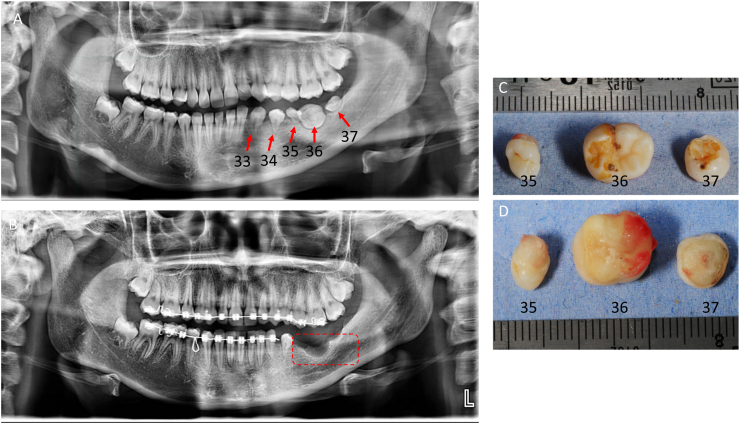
Panoramic radiograph showing abnormal development of teeth 33, 34, 35, 36, and 37, with notable defects in enamel and dentin (A). Following this radiographic assessment, the three most severely affected permanent teeth—one premolar (35) and 2 M (36 and 37)—were extracted for further analysis (B). Photographs of the extracted teeth show the occlusal aspect (C) and the roots aspect (D).
